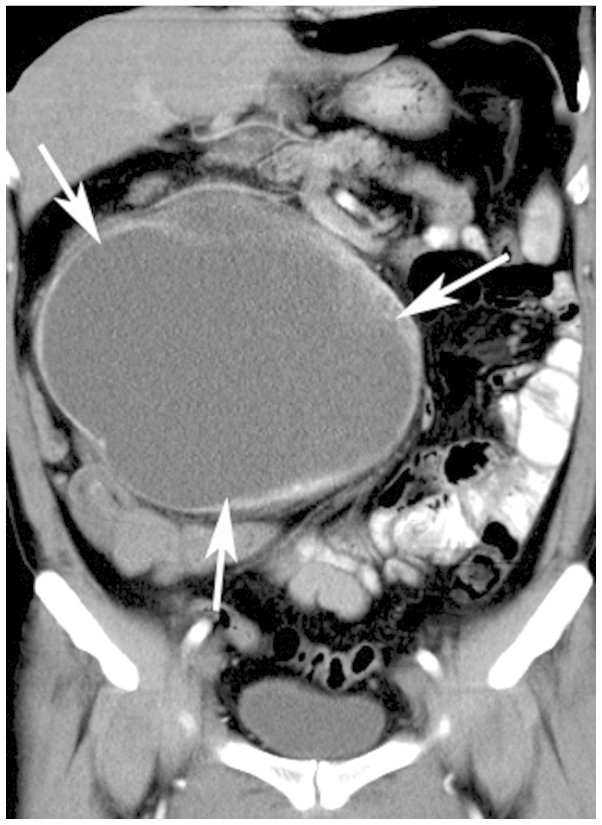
A 46-year-old male with a high-grade extragastrointestinal stromal tumor in the mesentery. Coronal contrast-enhanced computed tomography image showing a 19.3-cm cystic mass with markedly peripheral enhancement.
